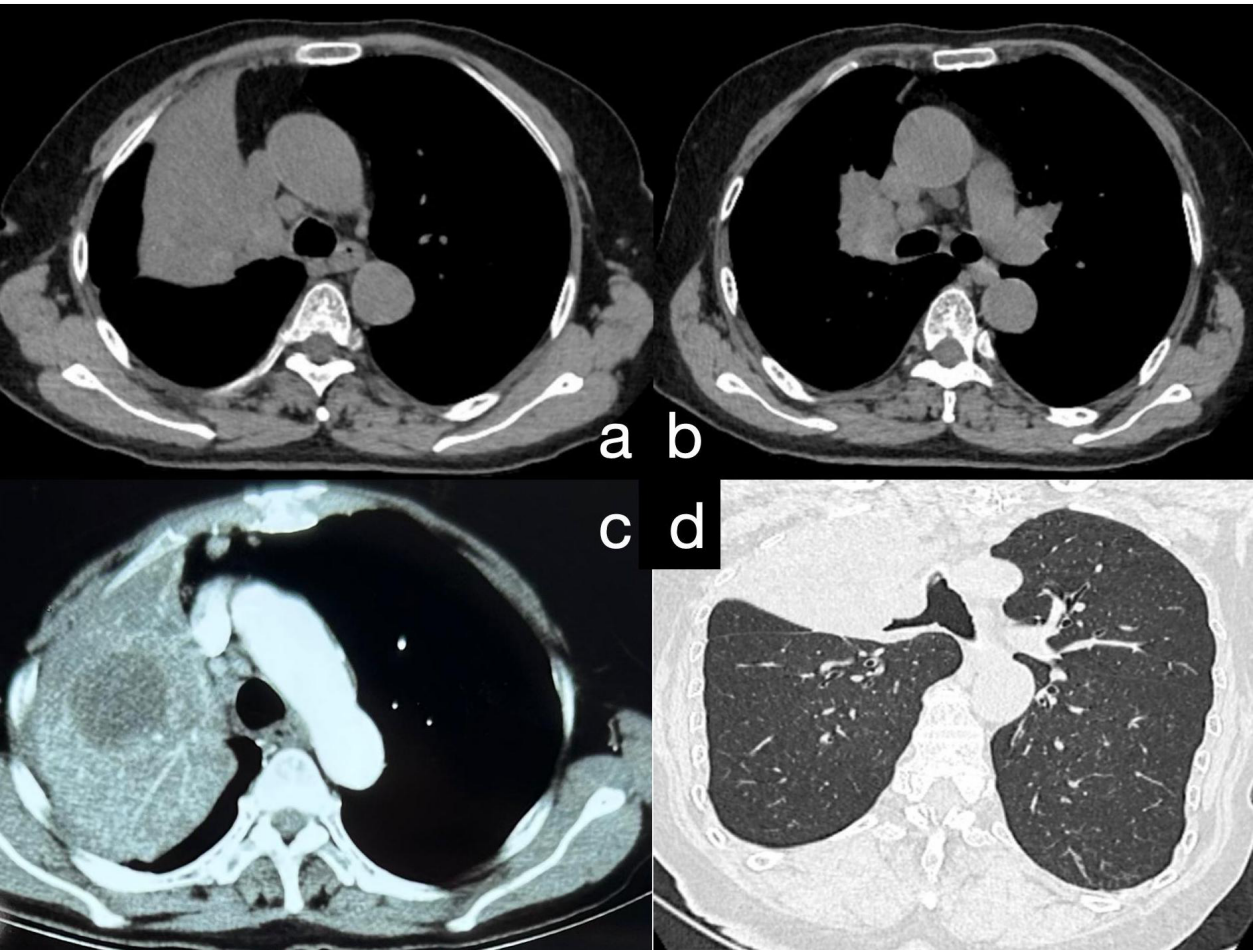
(A) Chest CT reveals a large area of consolidation in the upper lobe of the right lung. (B) Consolidation in the upper lobe of the right lung accompanied by multiple enlarged mediastinal lymph nodes. (C) Postcontrast chest CT demonstrates moderate enhancement in the consolidated area of the upper lobe of the right lung, with quasi-round, slightly hypodense lesions within the focus; the boundaries are still clear. (D) Following 3D reconstruction of the chest CT, bronchial narrowing and obstruction in the upper lobe of the right lung are evident.
